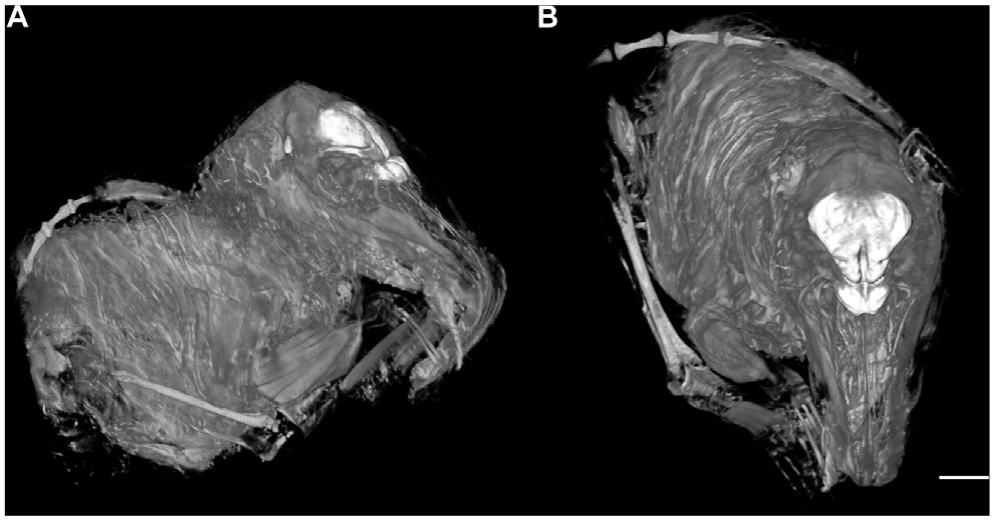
Maximum intensity projections of the *R. udzungwensis* MRI. Two views of the *R. udzungwensis* MRI visualized as maximum intensity projections with the brain highlighted in white. A) Antero-lateral oblique view. B) Superior view, scale bar = 5 cm.
